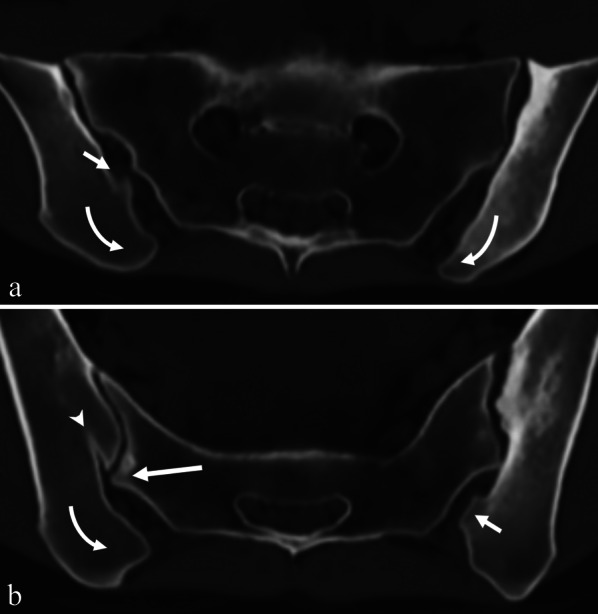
Multiple sacroiliac joint (SIJ) variants coexisting in one patient. Semi-axial (a) and axial (b) synthetic CT images of multiple coexisting variants in a 27-year-old woman. An accessory SIJ is visible posterior to the cartilaginous part of the SIJ on both sides (short arrow), as well as crescent iliac bony plate (curved arrow). Additionally, dysmorphic SIJ (long arrow) accompanied by degenerative subchondral cyst and bipartite iliac bony plate (arrowhead) are present on the right side. Also note erosions and marked sclerosis on the left side
